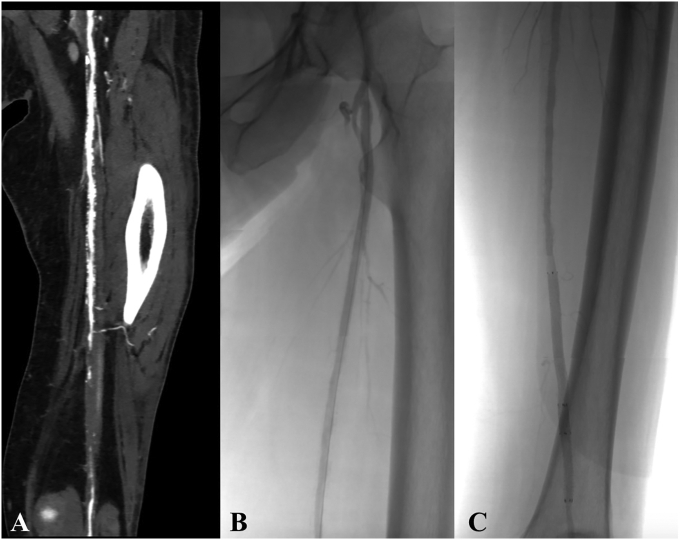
Preoperative CT-angiography showing atherosclerotic disease and occlusion of the left femoral artery (A); intraoperative angiography showing patency of superficial femoral artery after PTA with eluting ballon (B) and Stenting (C).
